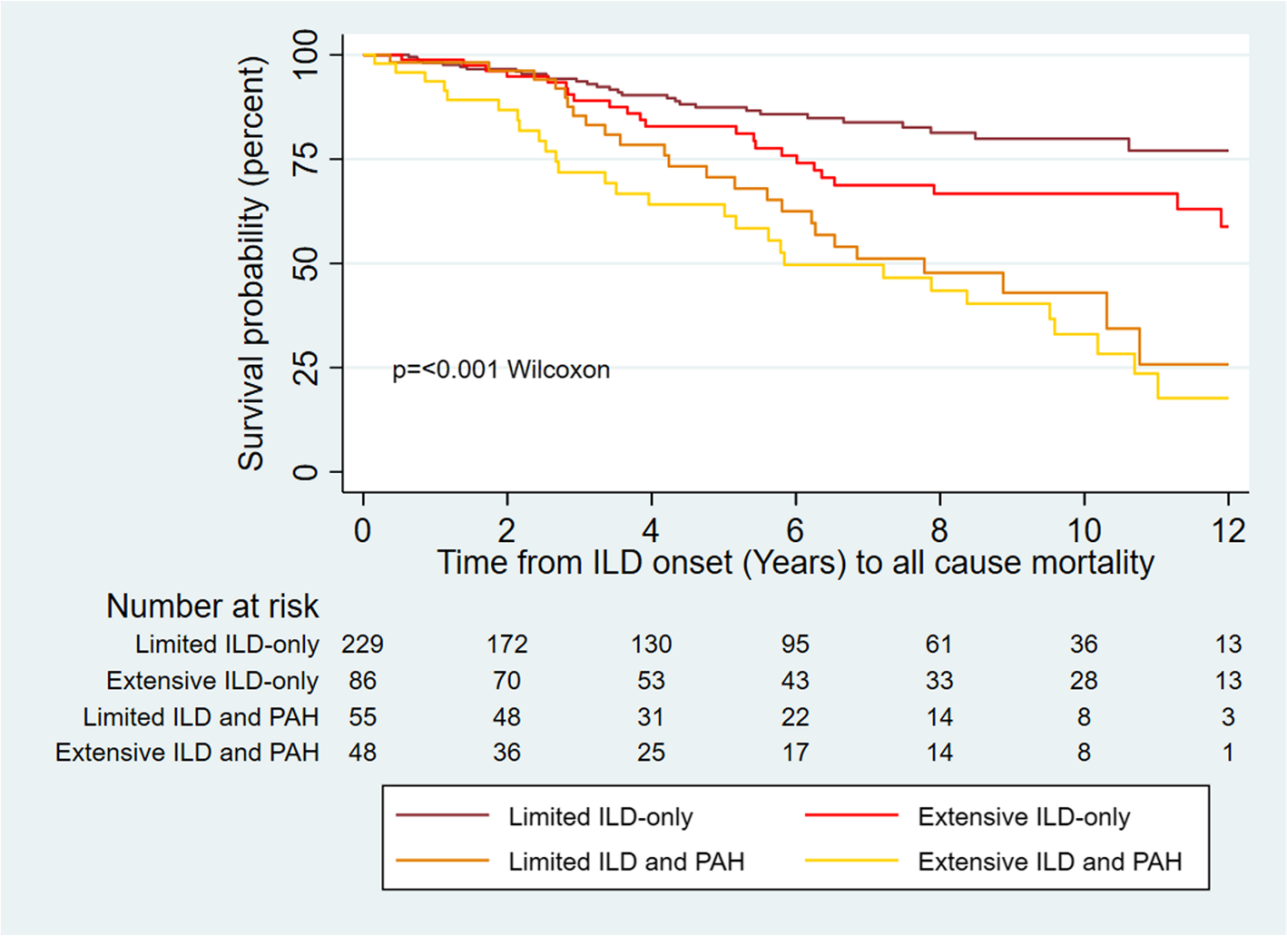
All-cause mortality from ILD onset in those with ILD. ILD, interstitial lung disease; PAH, pulmonary arterial hypertension; SSc, systemic sclerosis. ILD severity is defined as limited if < 20% involvement on HRCT or 20‒30% HRCT involvement with FVC ≥ 70%, or extensive if > 30% HRCT involvement, or 20‒30% HRCT involvement and FVC < 70%
